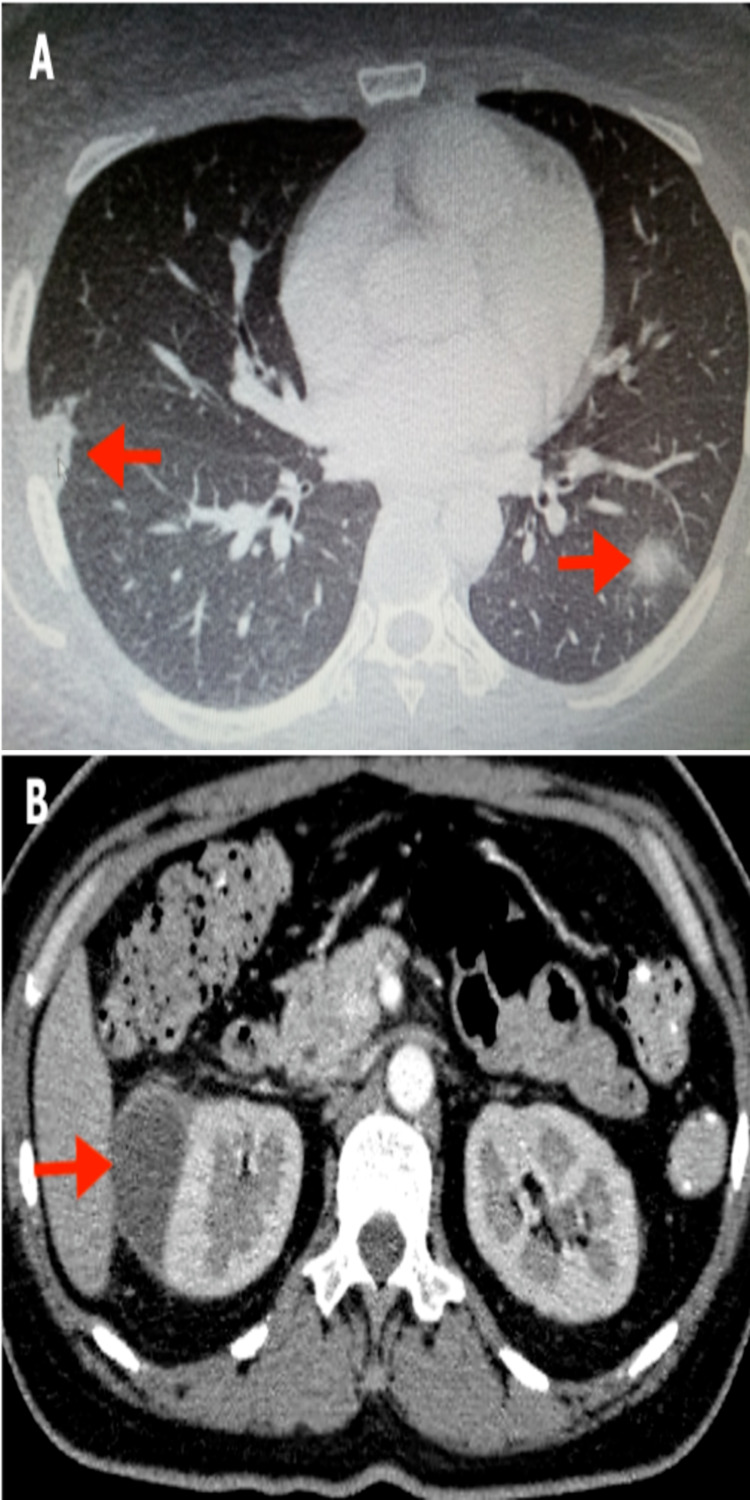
CECT thorax, abdomen, and pelvis shows (A) multiple cavitating lesions in both lungs suggestive of septic emboli; (B) right perirenal rim enhancing hypodense collection suggestive of an abscess CECT: contrast-enhanced computed tomography
